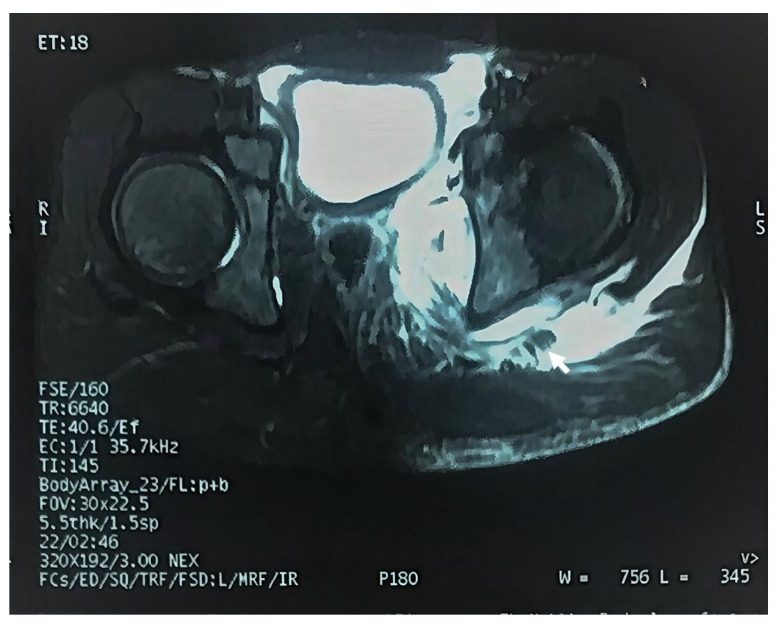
A contrast-enhanced MRI of the hip joint showing deep left pelvic inflammatory process (Arrow).
